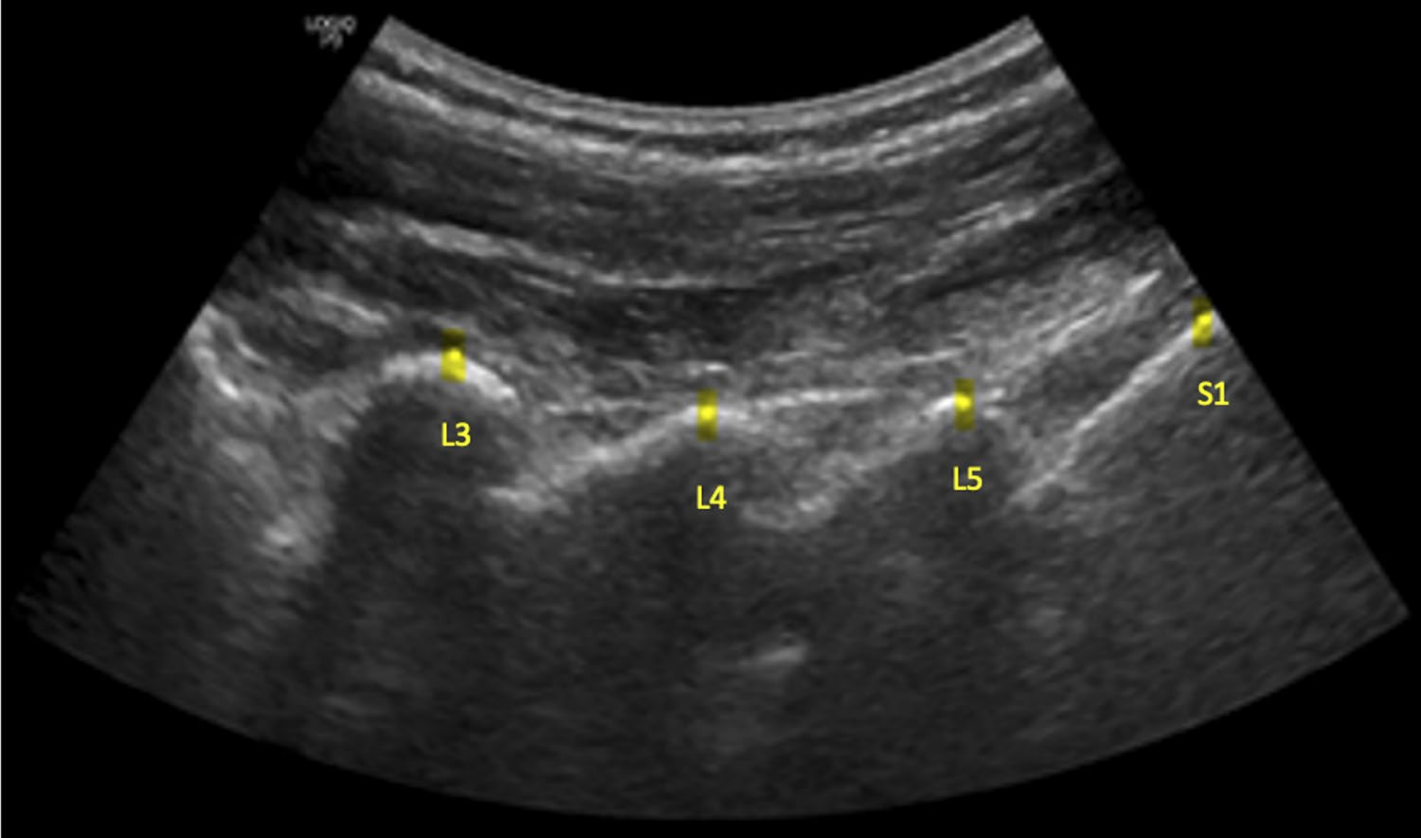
Ultrasound image annotation of the lumbar and sacral regions. Example of ultrasound image annotation of the lumbar region at the lumbo-sacral spine (L3, L4, L5, S1)
